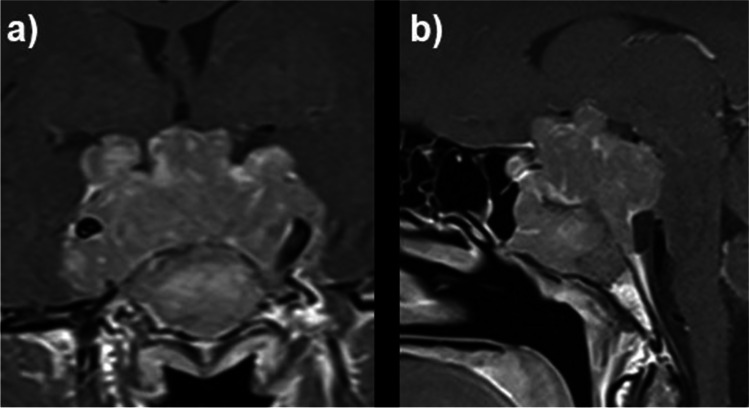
Coronal (a) and sagittal (b) MRI with contrast of a patient with a giant prolactinoma compressing the chiasm and invading the cavernous sinus on both sides, completely encasing the ICA (Knosp grade 4)
